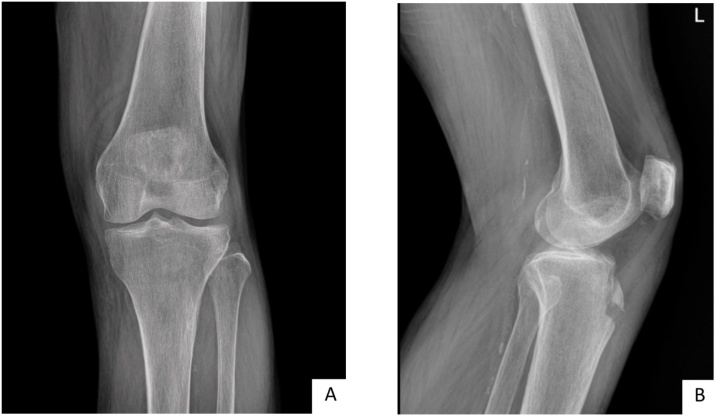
The initial radiographic imaging, (A) anteroposterior view of left knee, (B) lateral view of right knee.
